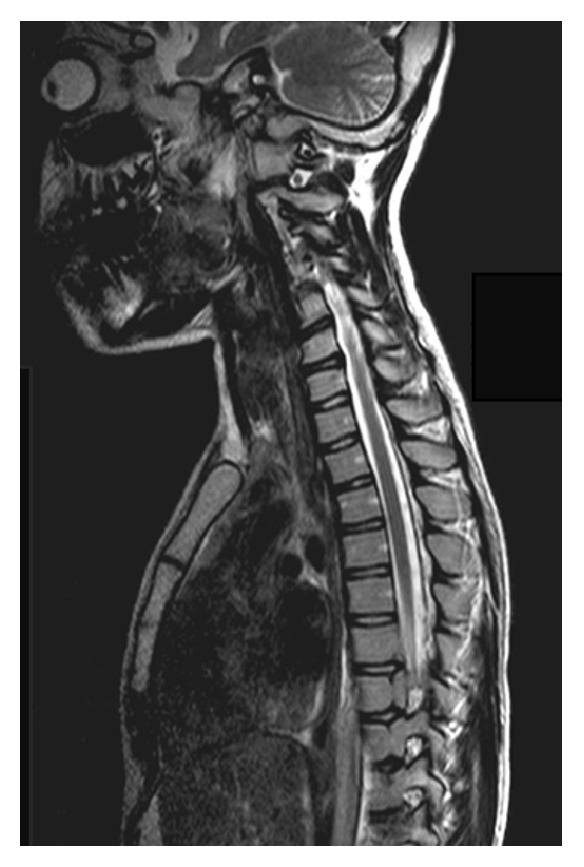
Rachis MRI detecting the presence of GAG around the dens of the epistropheus and the anomalies of spinal canal's diameter.
